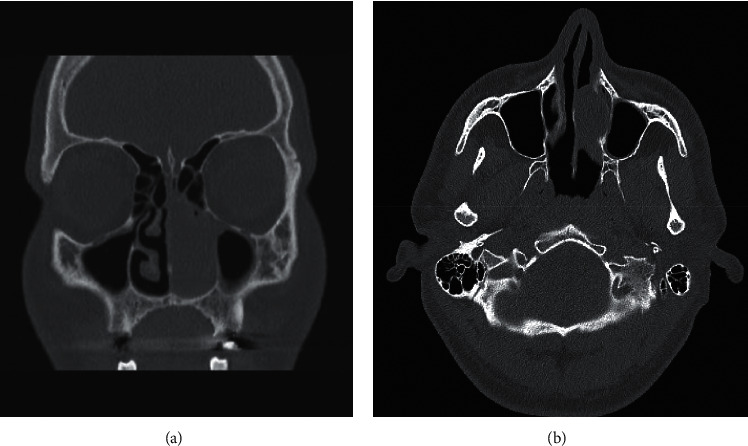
CT scan of the paranasal sinuses with contrast. The coronal and axial section shows a left anterior nasal mass.
